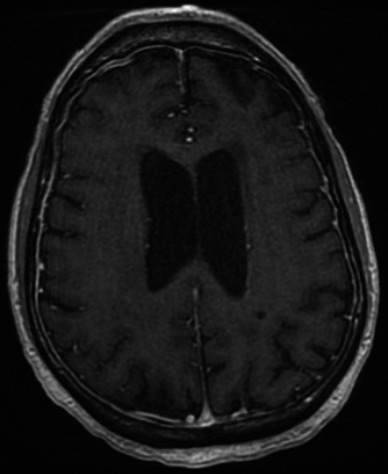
Axial image from MRI of the brain demonstrating generalized smooth pachymeningeal thickening and enhancement secondary to lymphomatous infiltration.
